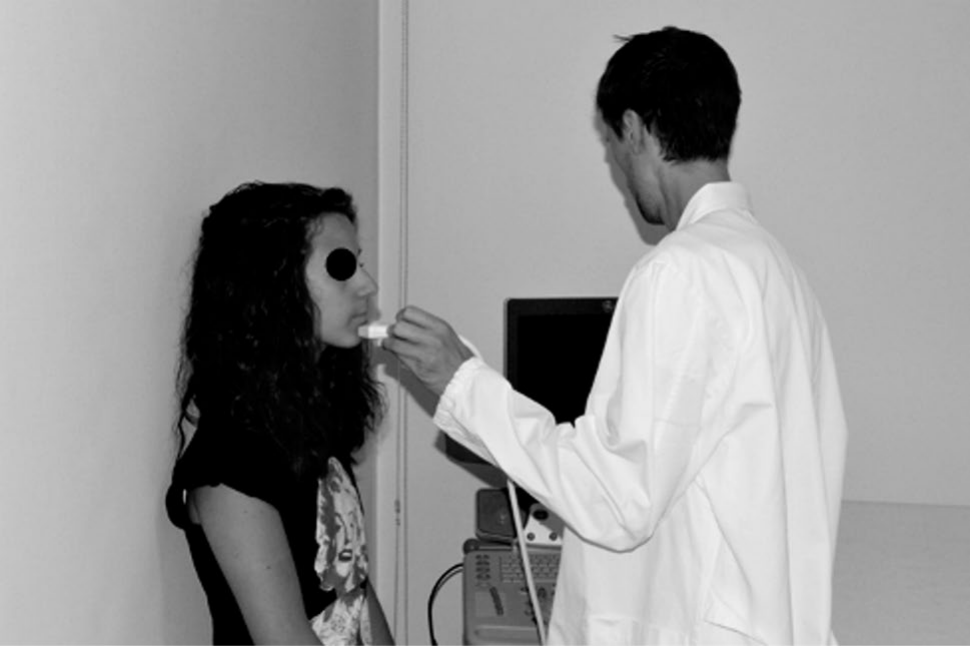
Ultrasonographic soft tissue thickness measurement
